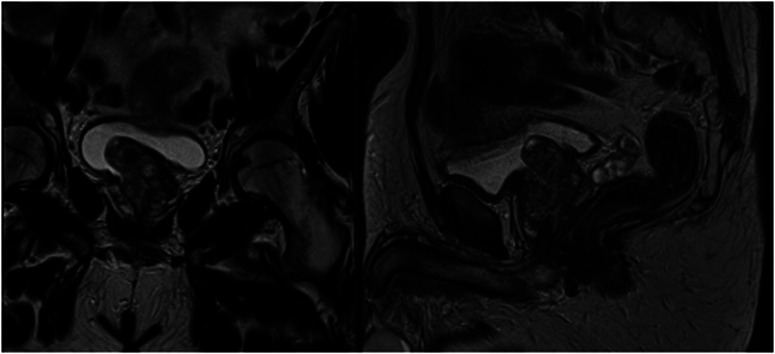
Intravesical protrusion of the prostate demonstrated on T2-weighted coronal and sagittal magnetic resonance image.
